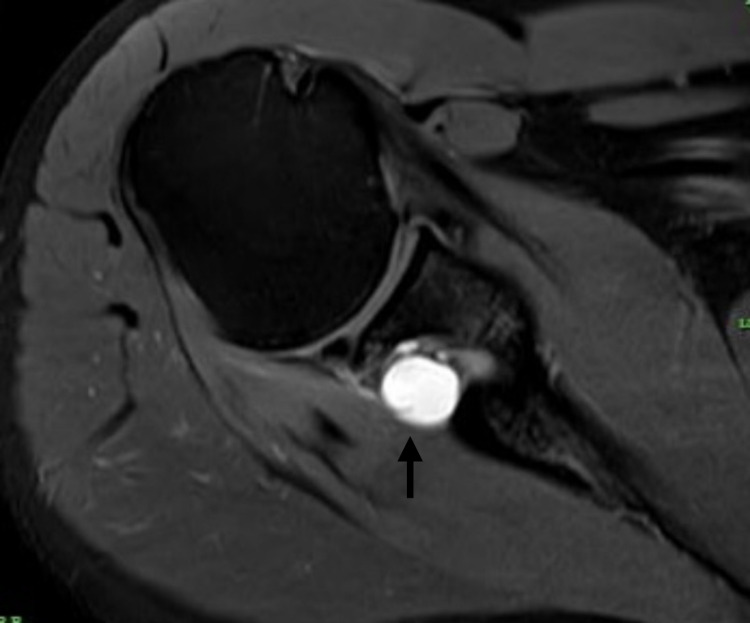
Axial cut of the right shoulder MRI showing spinoglenoid cyst (arrow)
